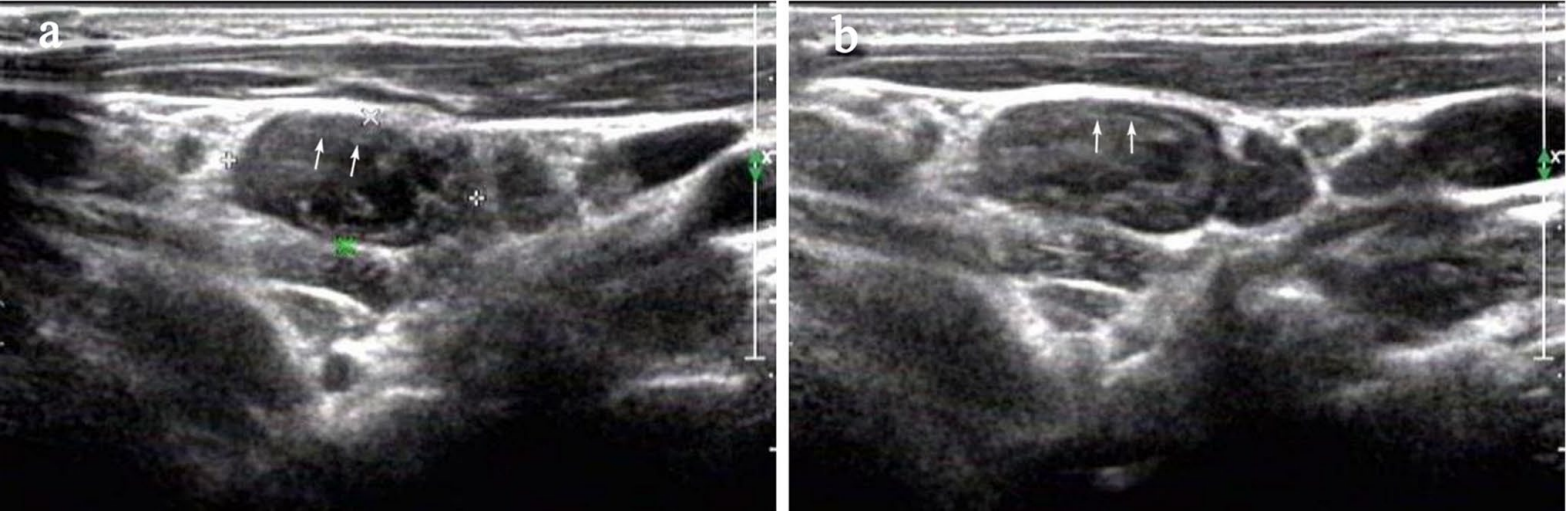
Cervical ultrasonography of lymph nodes (LNs) in a boy with tuberculous lymphadenitis. a Sonograms of LNs showing an echogenic thin layer with an irregular margin within the node, deep to the peripheral margin (arrows); b an echogenic thin layer in the peripheral margin within another node is shown (arrows)
